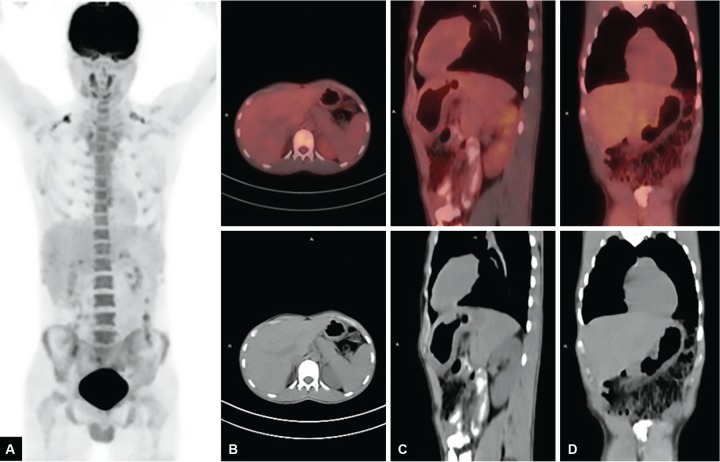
Follow-up F-18 FDG PET/CT images [maximum intensity projection (A), axial (B), sagittal (C), and coronal (D)] exhibited complete response to ATT with no residual disease
